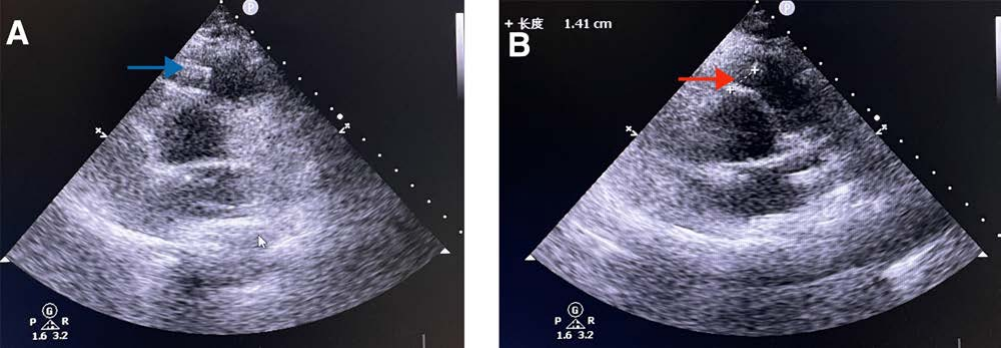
Transthoracic color short axis section of the great artery. (A) Location of the sheath in the right ventricular outflow tract (blue arrow). (B) The nearest distance from the sheath to the right coronary sinus is 1.41 cm (red arrow).
